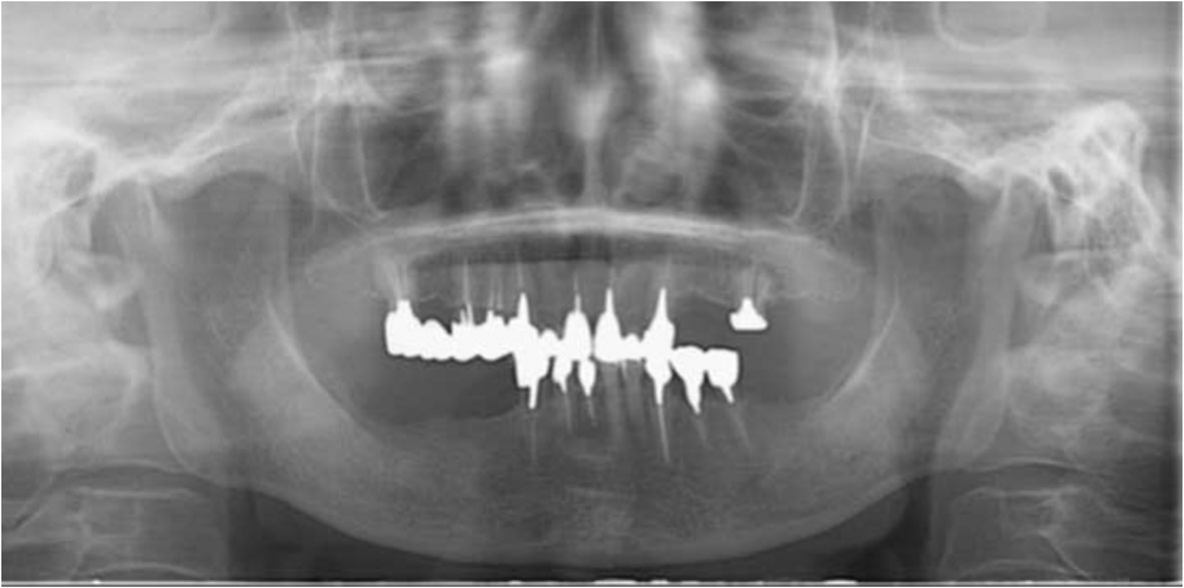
Panoramic radiograph obtained at the first examination. Only apical periodontitis of the left upper central incisor was observed
